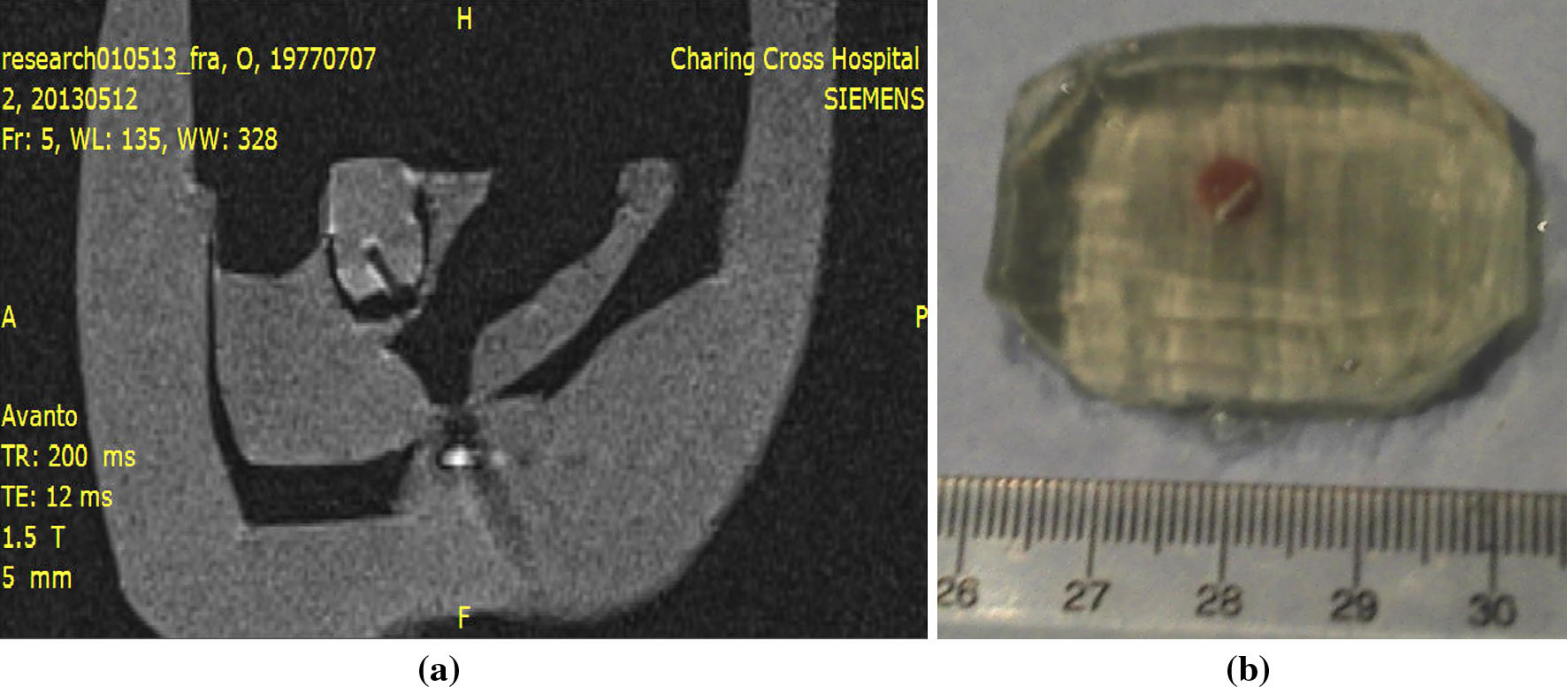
Targeting results: a sagittal MR image after firing; b photo of the actual target used in the experiment, with the needle track visible
